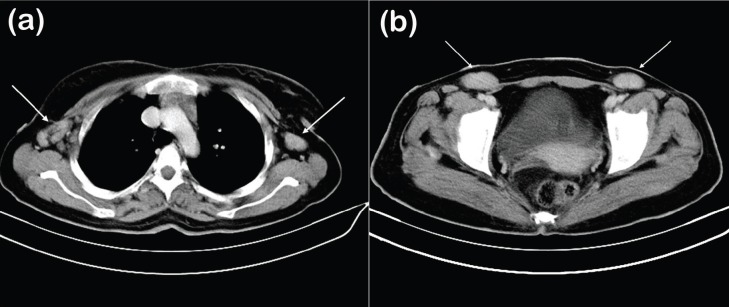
Contrast Enhanced Axial Computed Tomographic Images showed (a) bilateral enlarged axillary lymph node (white arrows) (b) Bilateral enlarged inguinal lymph nodes (white arrows).
